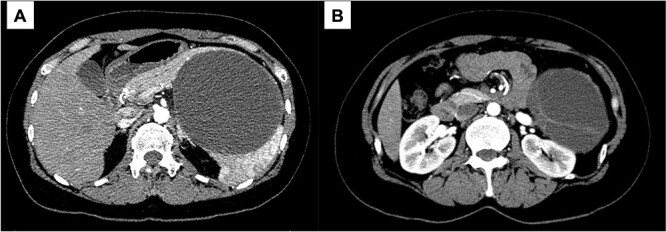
Contrast-enhanced CT; (A) the large cystic lesion in the pancreatic tail has displaced the stomach and the body of the pancreas; (B) the wall and septum show enhancement.
